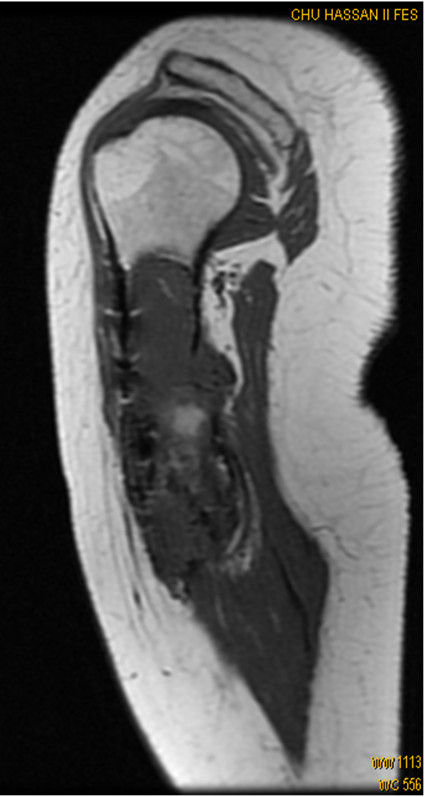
Sagittal T1 - weighted MR image after 3 cycles of chemotherapy shows partial decrease in tumor volume.
